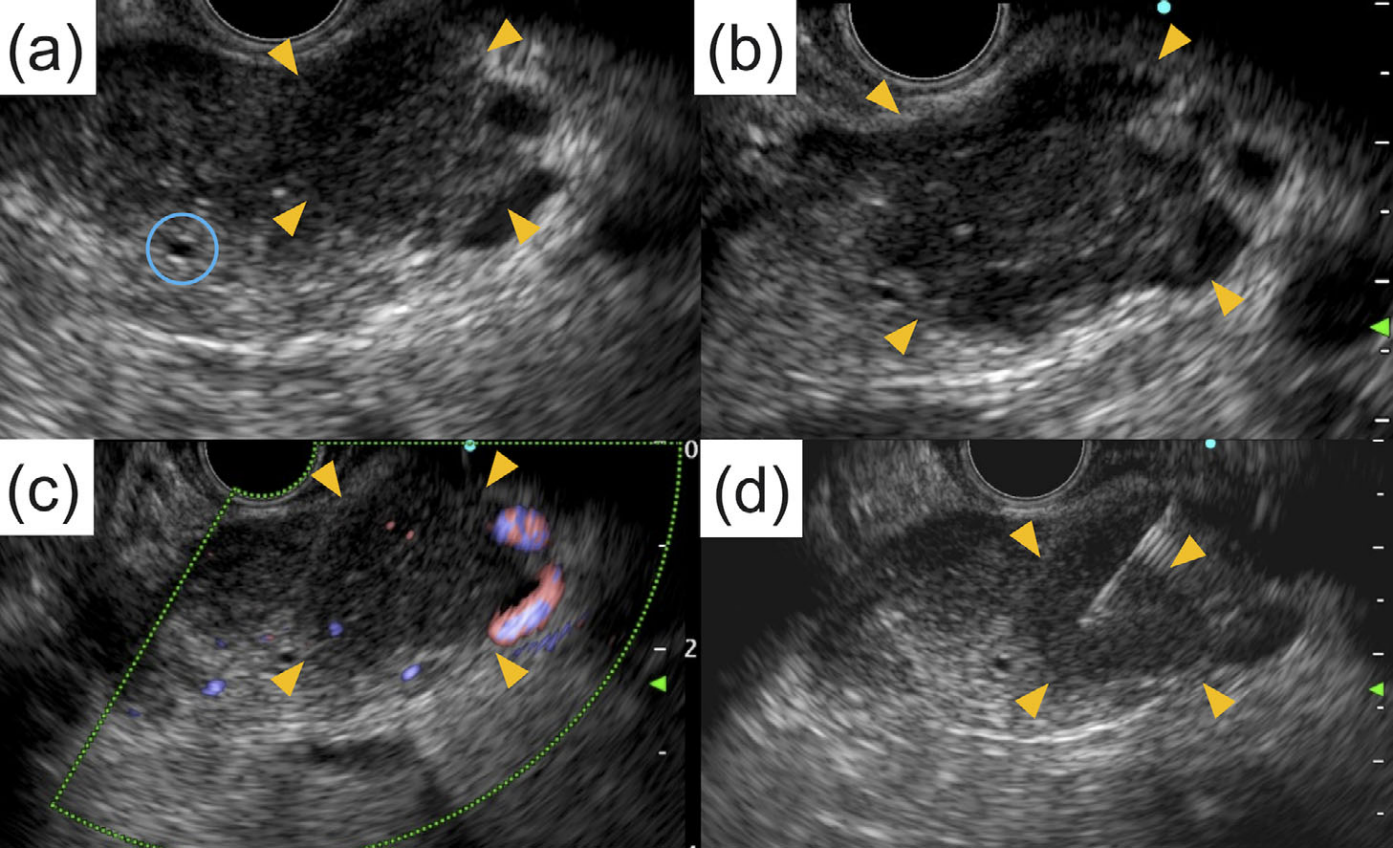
(a, b) Endoscopic ultrasound image (tissue harmonic echo imaging, penetration mode). A well‐defined hypoechoic mass was observed in the pancreatic tail (orange arrowheads). The tumor margins had an irregular morphology and were clearly demarcated from the surrounding normal pancreatic parenchyma. Internal echoes were heterogeneous. The main pancreatic duct was not involved in the lesion and was not dilated (blue circle). Lesion invasion into the gastric wall was not found. (c) H‐FLOW mode. Tumor blood flow was not extremely high. (d) An endoscopic ultrasound‐guided fine‐needle biopsy was performed.
